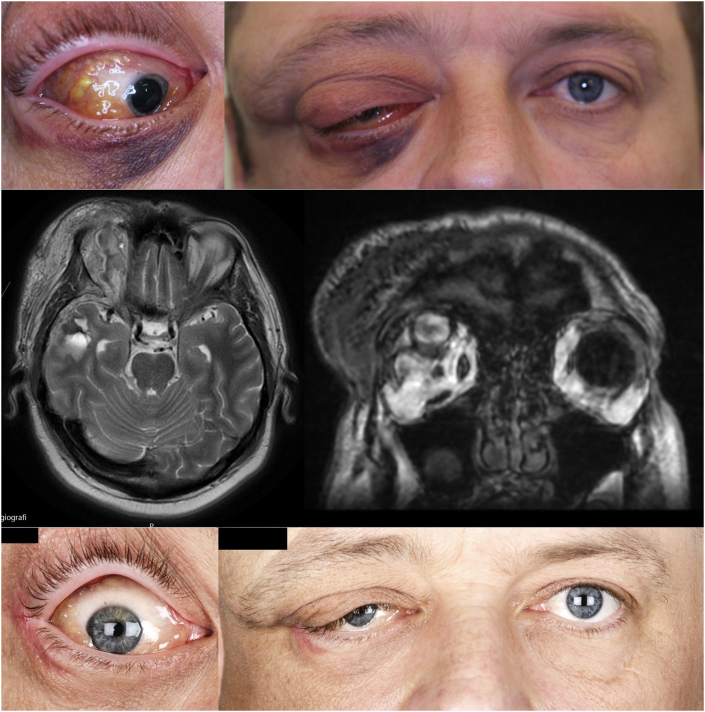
Case 3 – Patient with superior ophthalmic vein thrombosis and cavernous sinus thrombosis. A-B Photos at initial presentation. C-D Magnetic resonance imaging at initial presentation showing enlarged superior ophthalmic vein, orbital congestion and enlarged ocular muscles E-F Follow-up 10 weeks later.
